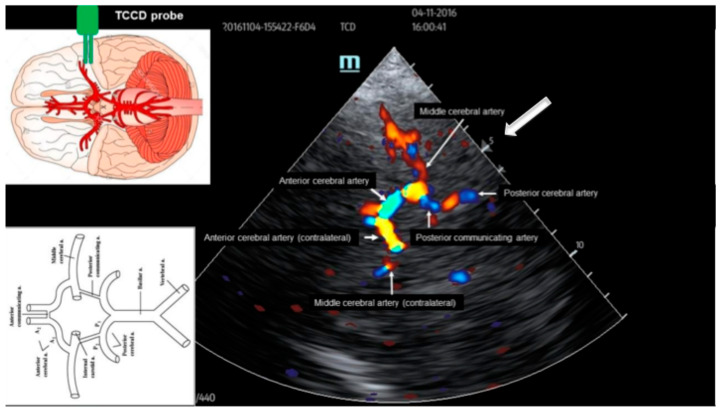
Anatomical orientation during transcranial colour-coded assessment of the circle of Willis. TCCD indicates transcranial colour-coded duplex. White arrow indicates the focus of the ultrasound beam during the investigation.
